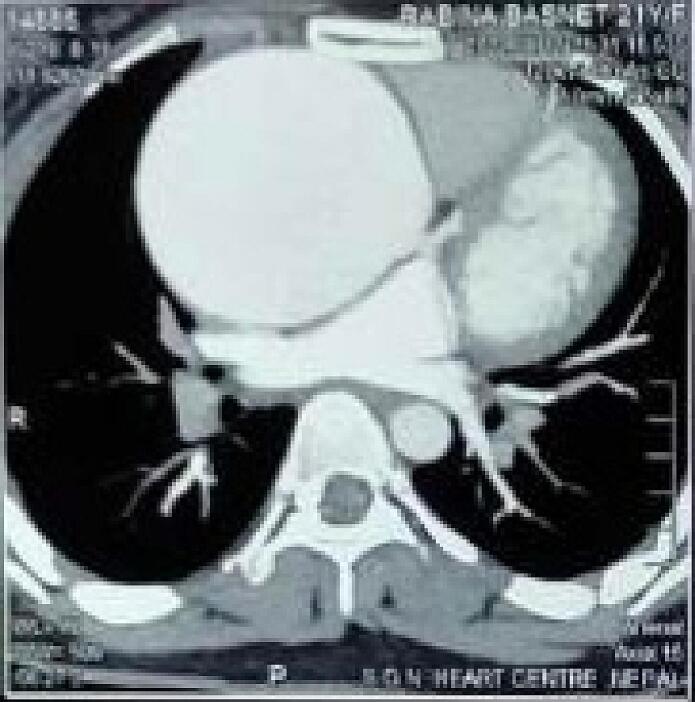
Computed tomography (CT) angiography. Axial view showing cross-section of the aneurysm at the level of ascending aorta.
